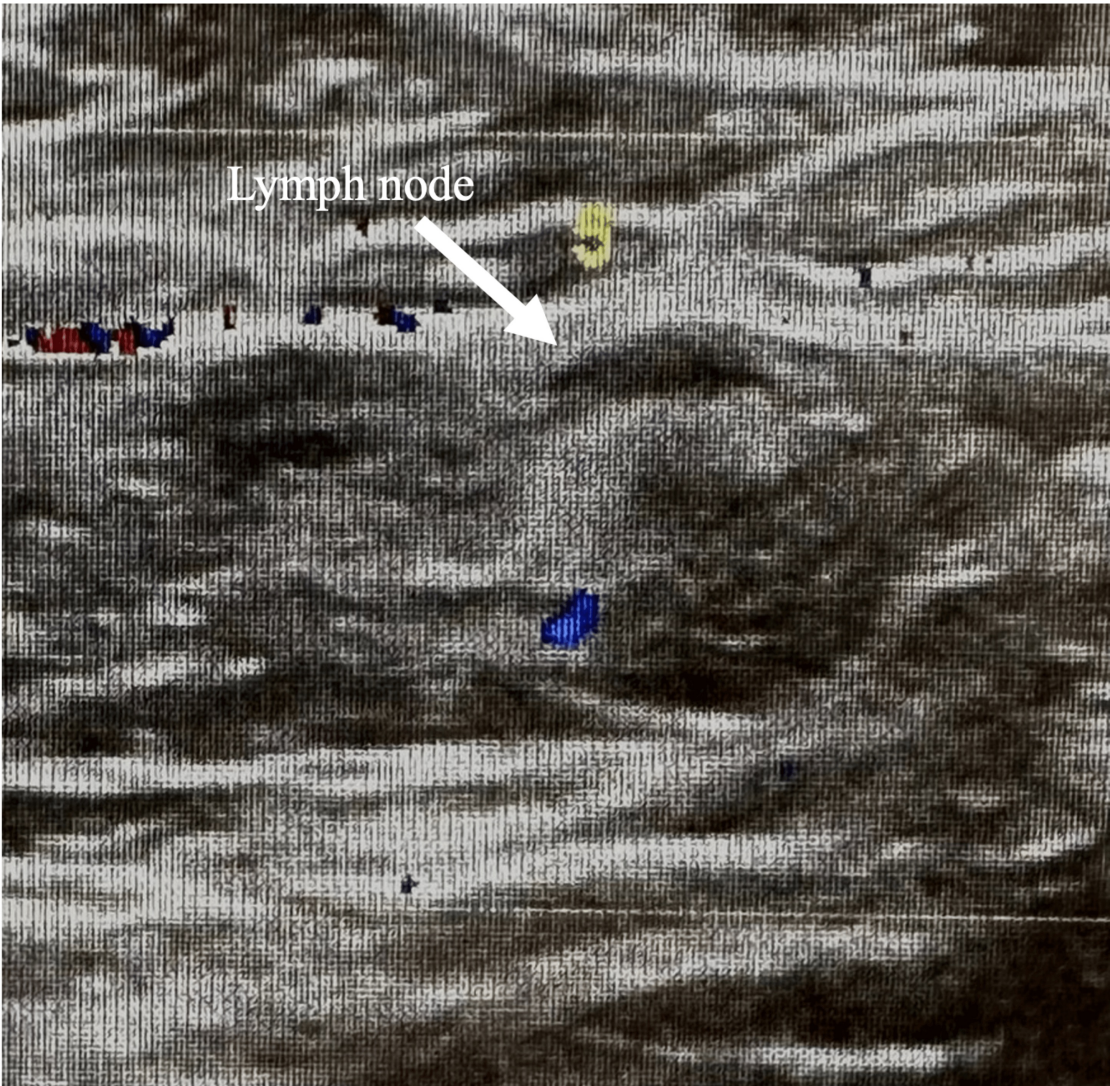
Ultrasound of left axilla shows thickened cortical margin to lymph node, suggesting malignancy.
